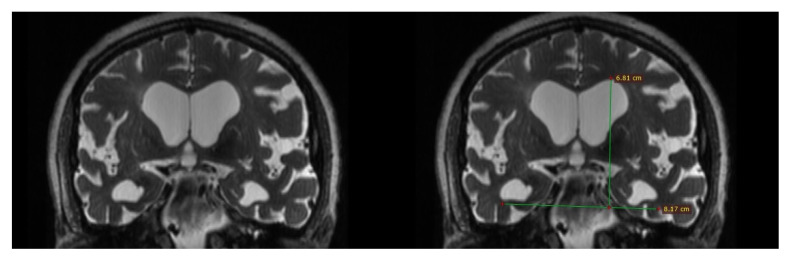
Reconstructed image in coronal plane created from 2 mm thin section T2-weighted images depict enlarged Sylvian fissures in a 74-year-old patient with iNPH. The new index value in this case was calculated as 1.19.
